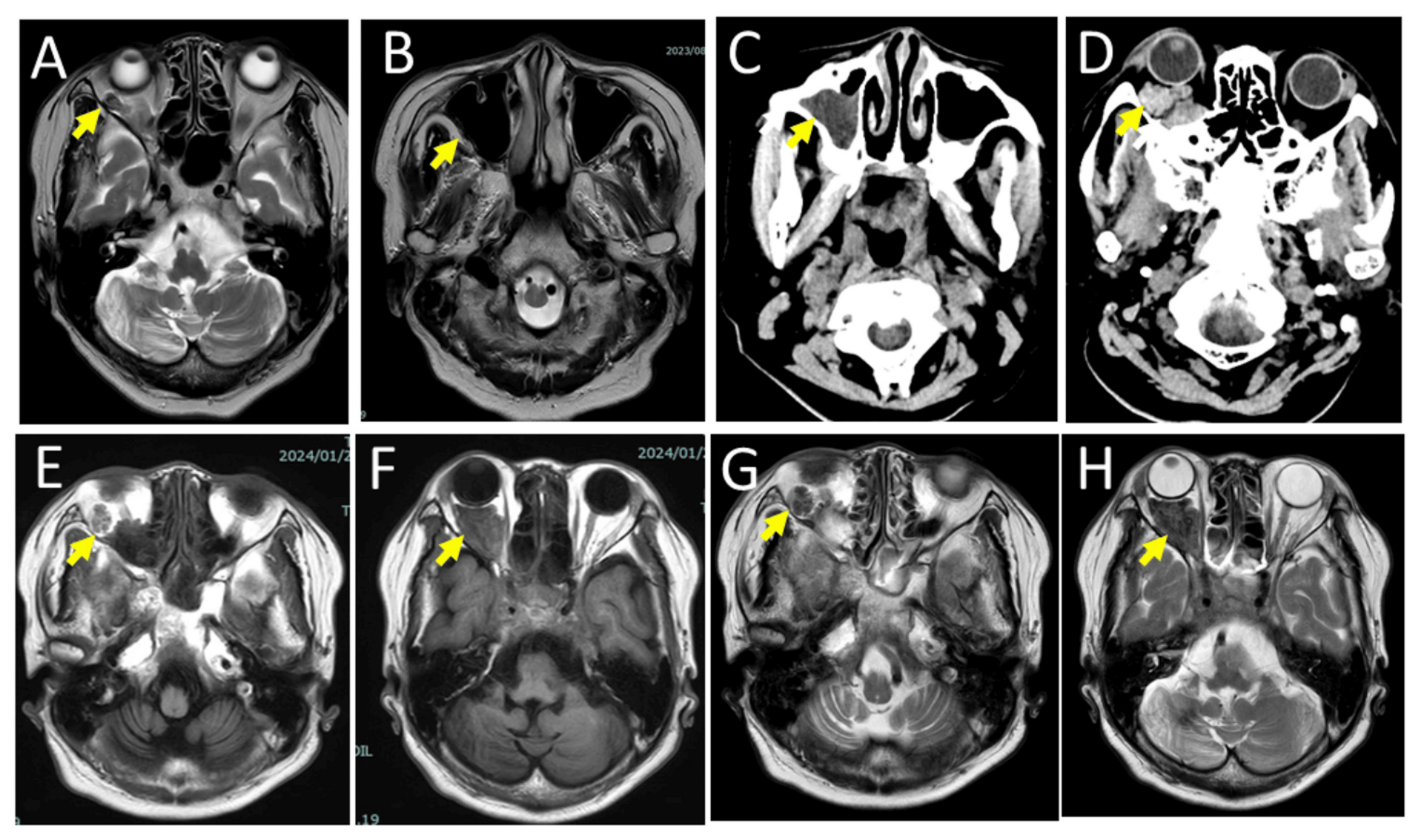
Magnetic resonance imaging half a year previously, computed tomography scans at the initial visit, and magnetic resonance imaging on the next day at referral. Incidental detection of right orbital vascular (venous) malformation (arrow, A) and the absence of right maxillary sinusitis (arrow, B) on T2-weighted magnetic resonance images half a year previously. Right maxillary sinusitis (arrow, C) and right orbital mass (arrow, D) with proptosis on emergency computed tomography scans at the initial visit to a regional hospital. Right orbital retrobulbar mass (arrows, F, H) in connection with a vascular malformation (arrows, E, G) in T1-weighted (E, F) and T2-weighted (G, H) axial images, next day at a referral hospital.
